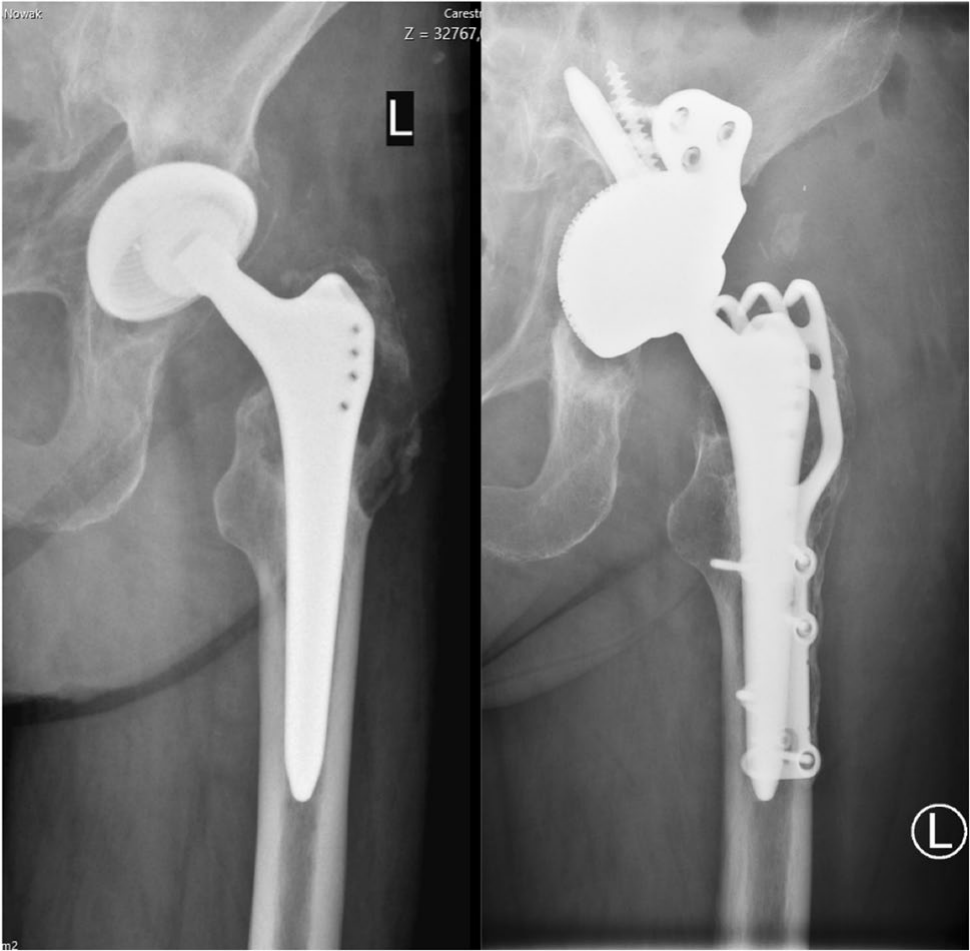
Pre- and post-operative frontal view radiographs of a female patient with marked osteolysis due to polyethylene wear. The synovial fluid calprotectin level measured intra-operatively was 32 mg/dl
